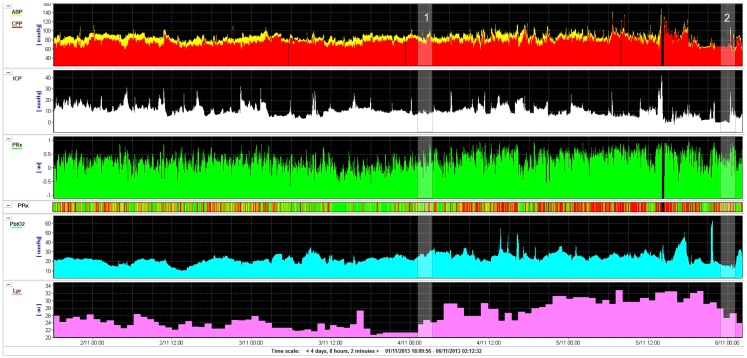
Example of monitoring (for 4 days) in a road traffic accident victim (aged 22 years): intracranial pressure (ICP), cerebral perfusion pressure (CPP), pressure reactivity index (PRx), brain tissue oxygenation (PbtO_2_), and lactate/pyruvate ratio (LPR). Initial GCS was 13 and deteriorated quickly to 7. Patient had never daily averaged ICP above 20 mmHg, CPP was above 70 mmHg all the time. On day 2 after injury, pressure reactivity (PRx) deteriorated (marker 1, this can be also read by “risk plot,” where PRx is color coded: green–good reactivity, red–deteriorated reactivity) and lactate/pyruvate ratio exceeded 25 and 30 later. On day 4, the patient improved (good CT scan), lactate/pyruvate ratio decreased, and PRx improved (marker 2). The patient was weaned uneventfully from the ventilator and successfully extubated shortly afterward. This example shows that lactate/pyruvate ratio and PRx may be markers of deterioration, adding clinical information independently of ICP and CPP.
